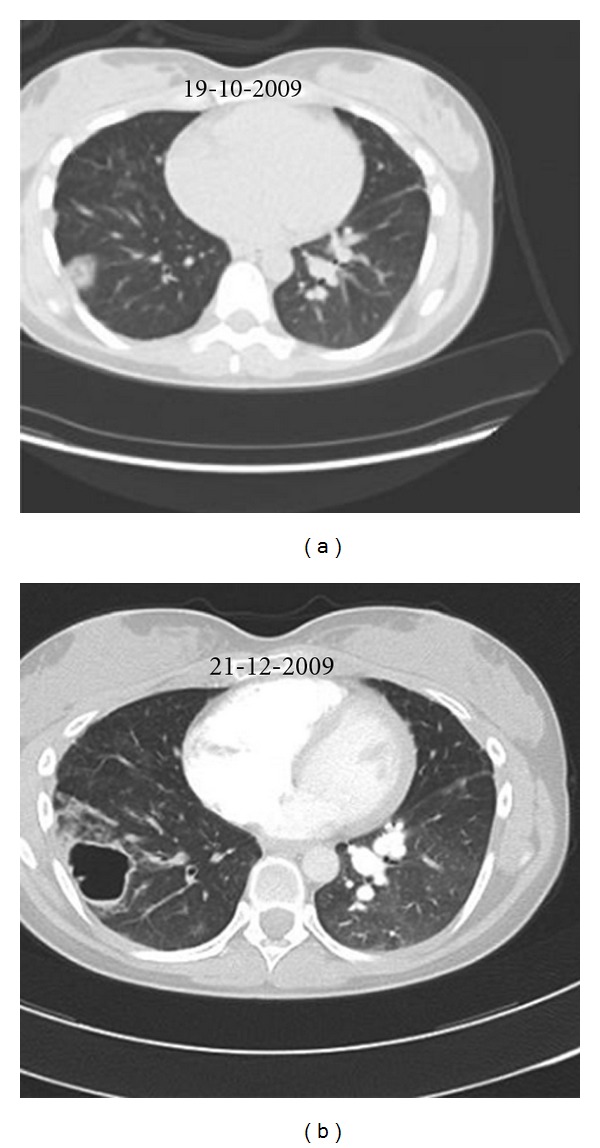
CT thorax shows an initial lung infiltrate (a) evolving into a large thin-walled cavitary lesion in the right lower lobe (b).
